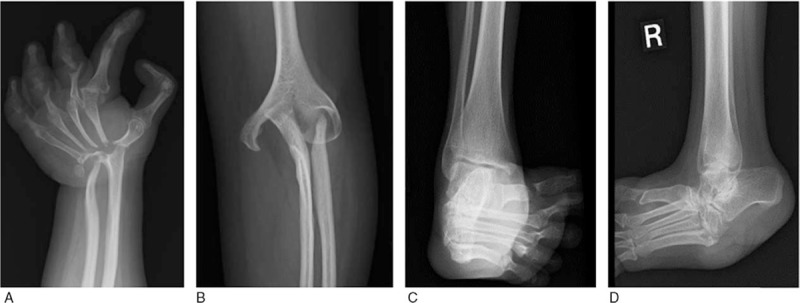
X-ray images of (A) hand joints; (B) elbow joint; (C) ankle joint; (D) foot.
